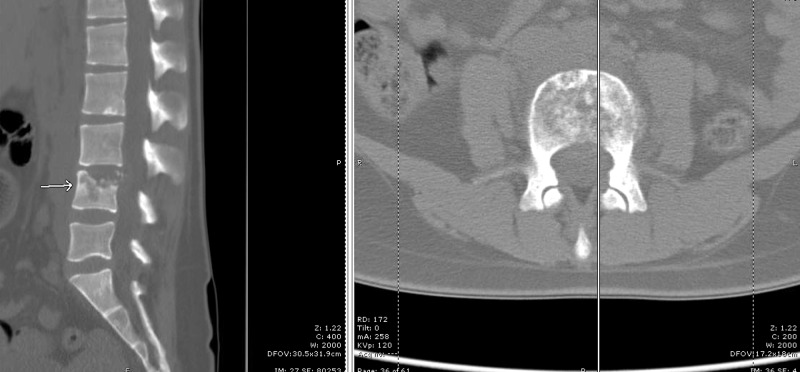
CT lumbar spine Sagittal (left) and axial (right) views of computed tomography (CT) of the lumbar spine showing lytic changes at the L4 vertebral body (arrow) and mild narrowing of the L3-4 disc space
